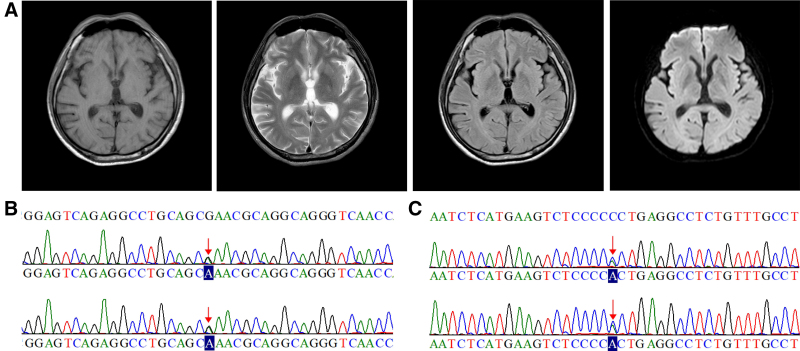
Brain MRI and *NPC1* gene sequencing results. (A) Brain MRI with diffusion-weighted imaging showed no obvious parenchymal abnormalities. (B) Sequencing results of the *NPC1* gene in the patient and his sister revealed 2 missense variants. Both individuals harbored a C > T substitution at position c.2932 in exon 20 of the *NPC1* gene (c.2932C > T), which resulted in an arginine-to-cysteine substitution at amino acid 978 (p.Arg978Cys, indicated by the arrow). (C) The patient also carried a G > T substitution at position c.2974 of the *NPC1* gene (c.2974G > T), leading to a glycine-to-tryptophan substitution at amino acid 992 (p.Gly992Trp, indicated by the arrow). MRI = magnetic resonance imaging.
